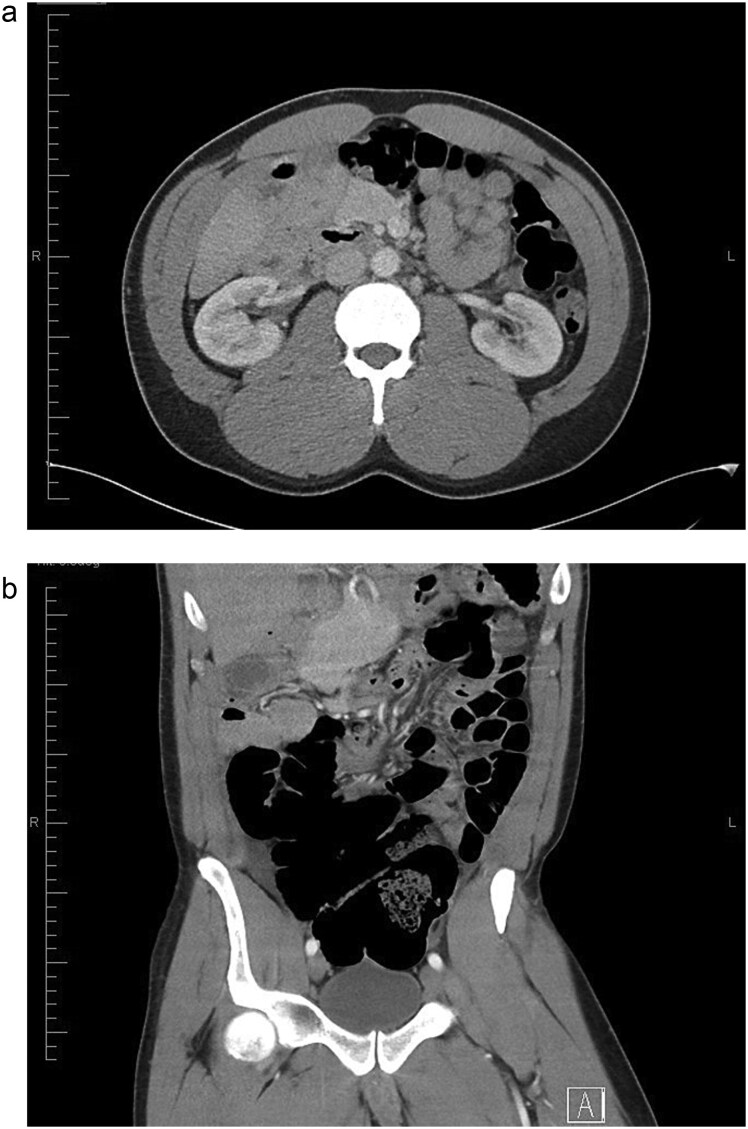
(a) Axial view of CT abdomen with contrast showing swirl sign i.e. swirling of bowel and mesentery; suggesting intestinal volvulus (superior to SMA and SMV. (b) Coronal view of CT abdomen with contrast showing swirl sign i.e. swirling of bowel and mesentery; suggesting intestinal volvulus.
